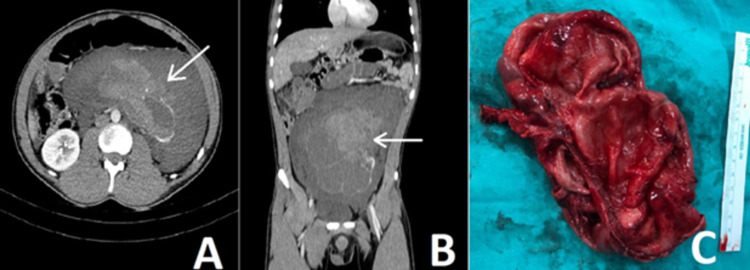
(A) Hematoma was observed at the intra-abdominal and left retroperitoneal areas in axial sections with a contrast-enhanced abdominal CT. The arrow shows a contrast extravasation consistent with active bleeding. (B) Coronal sections show hematoma that spreads to almost the entire abdomen and the arrow shows the contrast extravasation. (C) Nephrectomy material. The kidney has almost completely lost its parenchyma tissue.
